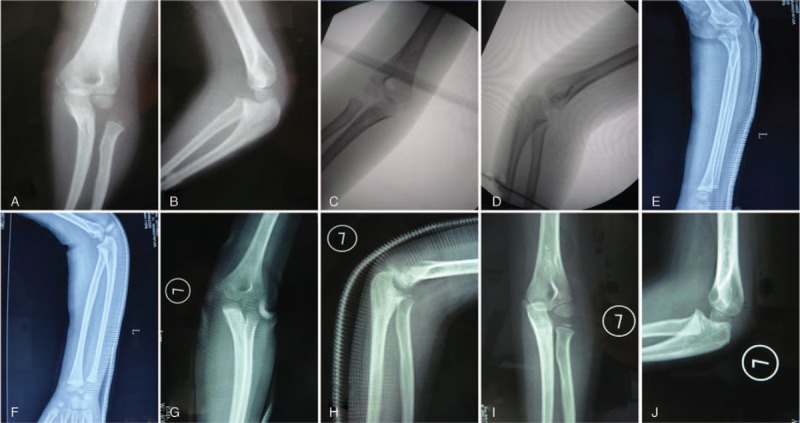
A 5-year-old girl presented 3 days after injury of her left elbow with initial type III Bado injury (A and B). She was treated with closed reduction of the ulna fracture and radial head, and application of a cast to immobilize the forearm (C–F). The cast was removed 6 weeks after the manipulation, and the girl was encouraged to begin movement of the elbow and gradually return to normal activities (G and H). X-ray images obtained 12 months after manipulation (I and J) showed maintenance of the reduction.
